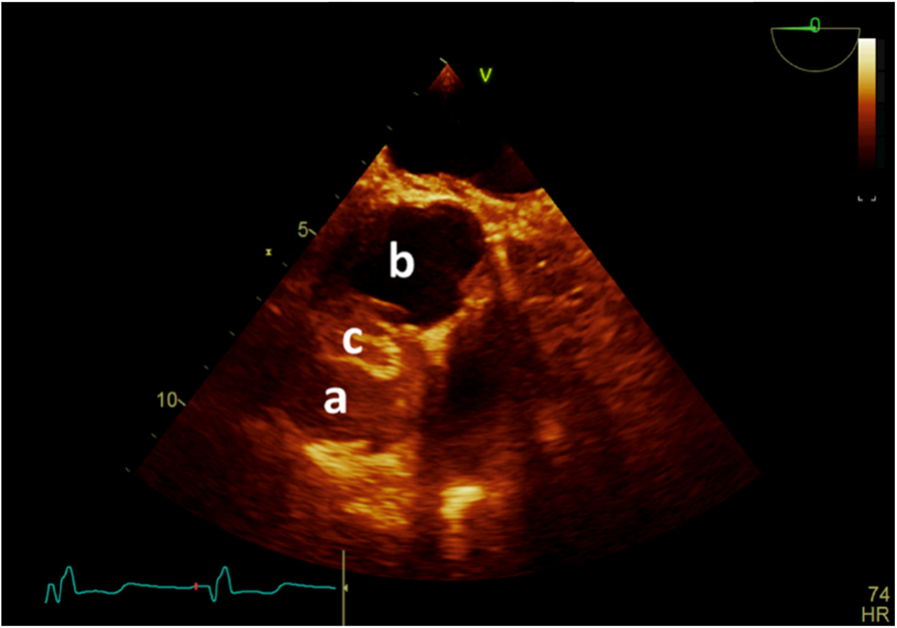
Inra-opperative Trans-esophageal echocardiography. Completed and released device with good position and no residue. A pseudoaneurysm, (B) ascending aorta, (C) ASD device occluder
